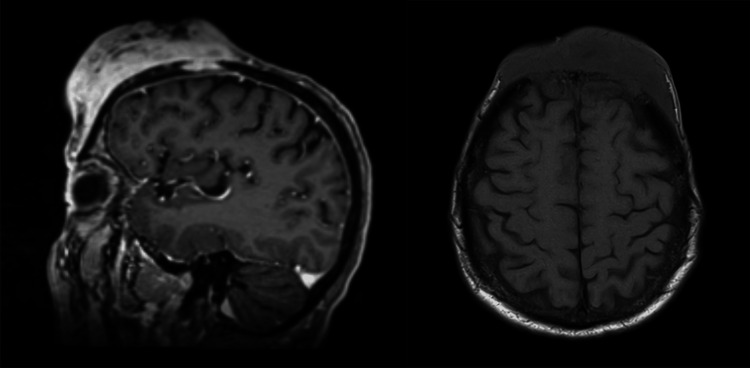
Sagittal and axial MRI views of the brain depicting a large fronto-parietal scalp mass with skull invasion and dural involvement
